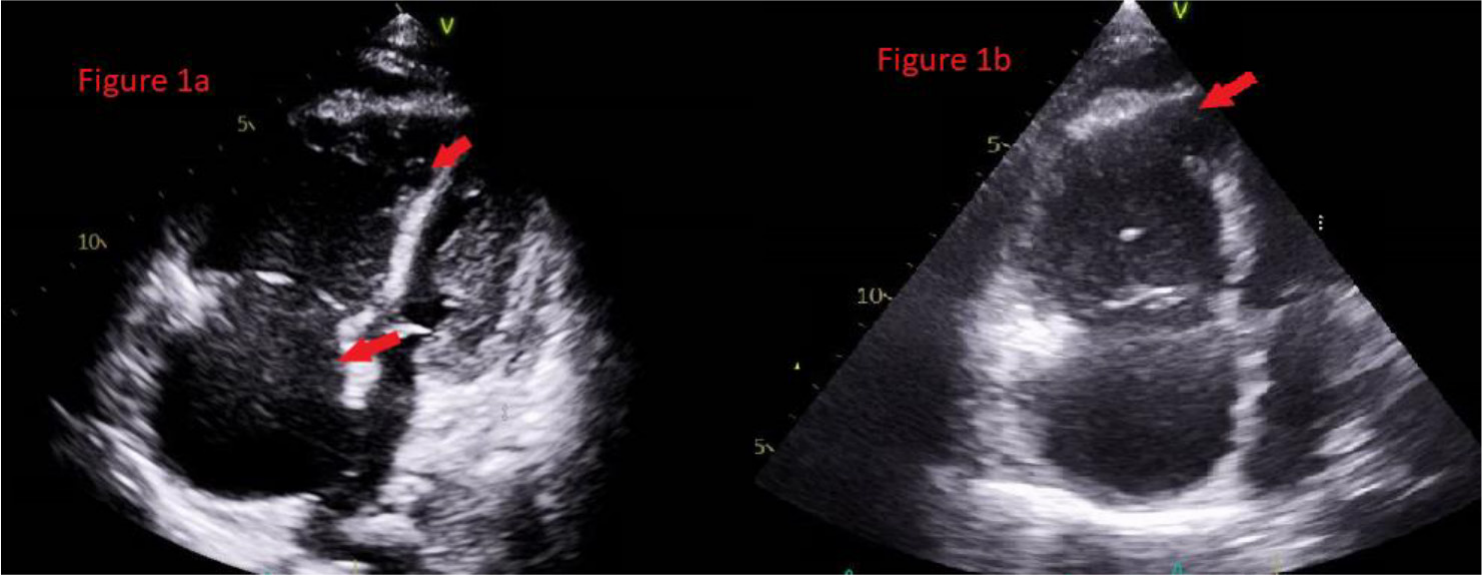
Transthoracic echocardiography of dilated atrium showing early forming Thrombus (a) and dilated right ventricle with McConnell’s sign (b).
